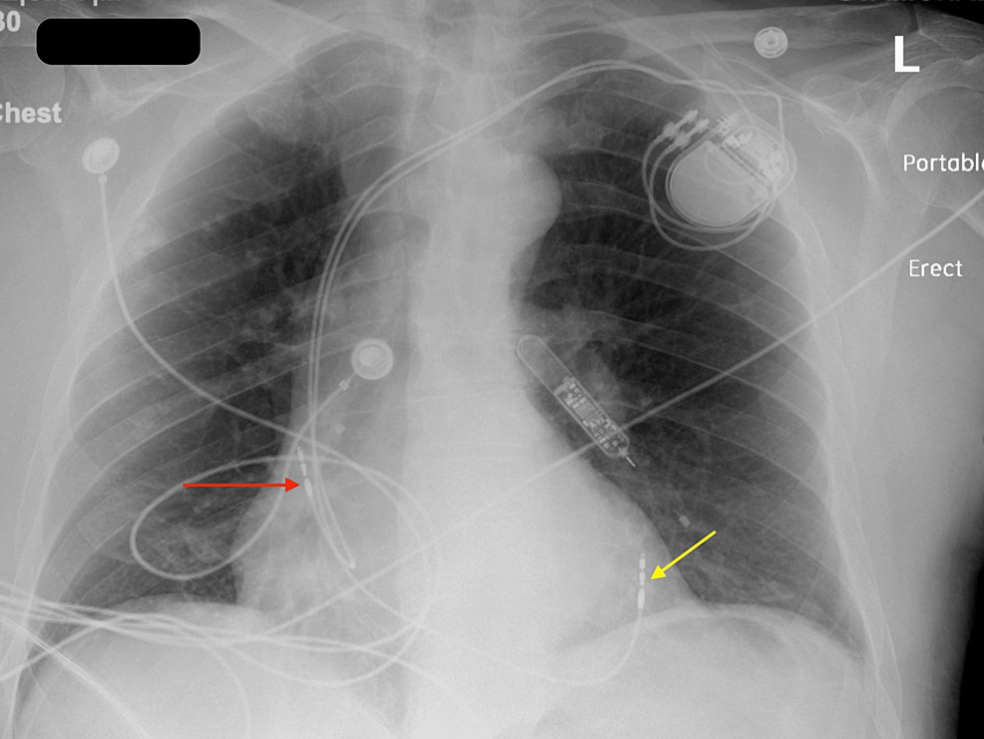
Chest X-ray PA view on the presentation Chest X-ray PA view on presentation shows the right atrial pacemaker lead in place (red arrow), and dislodgement and migration of the right ventricular pacemaker lead (yellow arrow).
